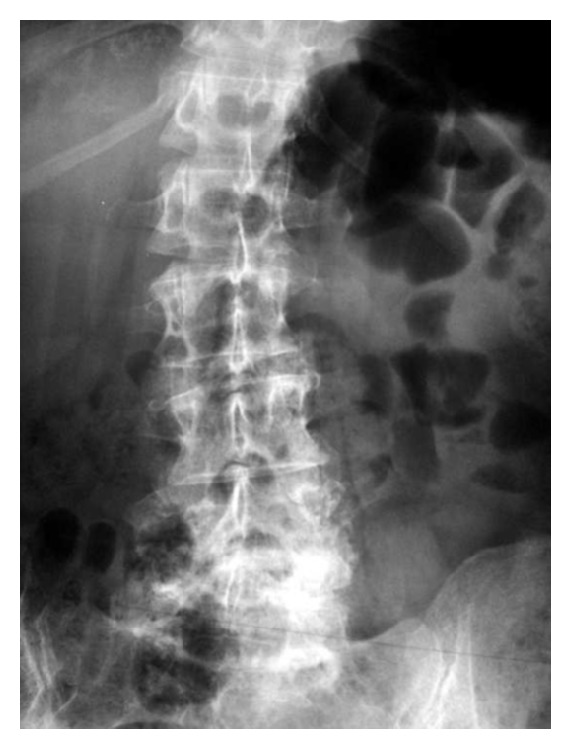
Anteroposterior radiograph shows leftward translation of L4 (Case 2).
